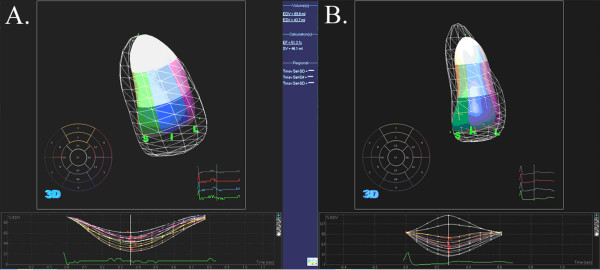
3D echocardiographic analysis of segmental left ventricular volume change. A and B. A represents data from a healthy subject and B represents data from AL subject. Top panel shows 16 segments analyzed (excludes apex) with solid cast representing endsystolic volume and surrounding grid cast representing beginning systole (or end-diastole) volume. The bottom panel shows the regional volume expressed in proportion to end-diastolic volume with time during the cardiac cycle. In these 2 examples, the dispersion of timing of endsystole is narrower in A compared to B.
